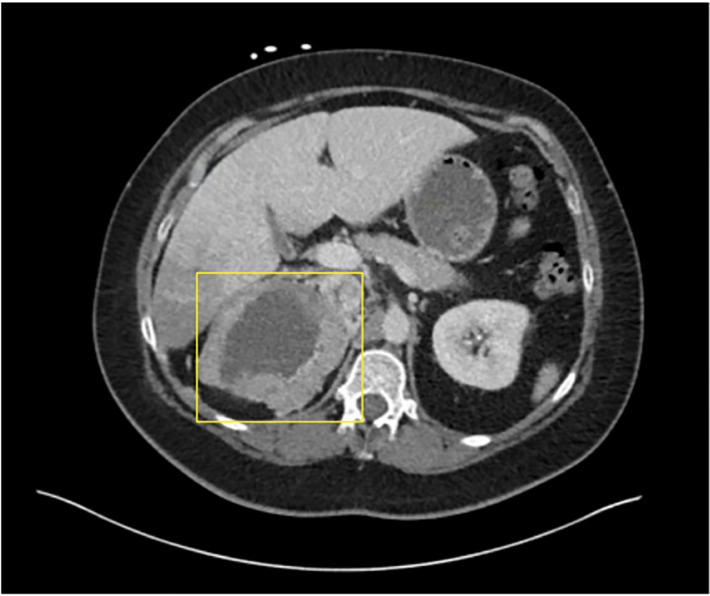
Axial computed tomography demonstrating cystic mass in right upper quadrant (yellow box).
